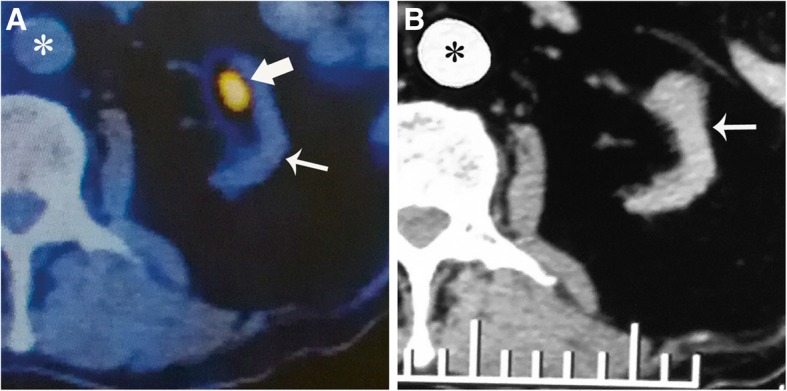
Positron emission tomography/computed tomography (PET-CT) and computed tomography (CT) images of the outer hospital. a PET-CT revealed a 1.4 cm intense FDG uptake (Standardize Uptake Values max: 4.7) lesion (wide arrow) at the medial portion of the left native kidney; b AS the same level of PET-CT, contrast-enhanced CT did not detect any solid lesion in the left native kidney (narrow arrow)
